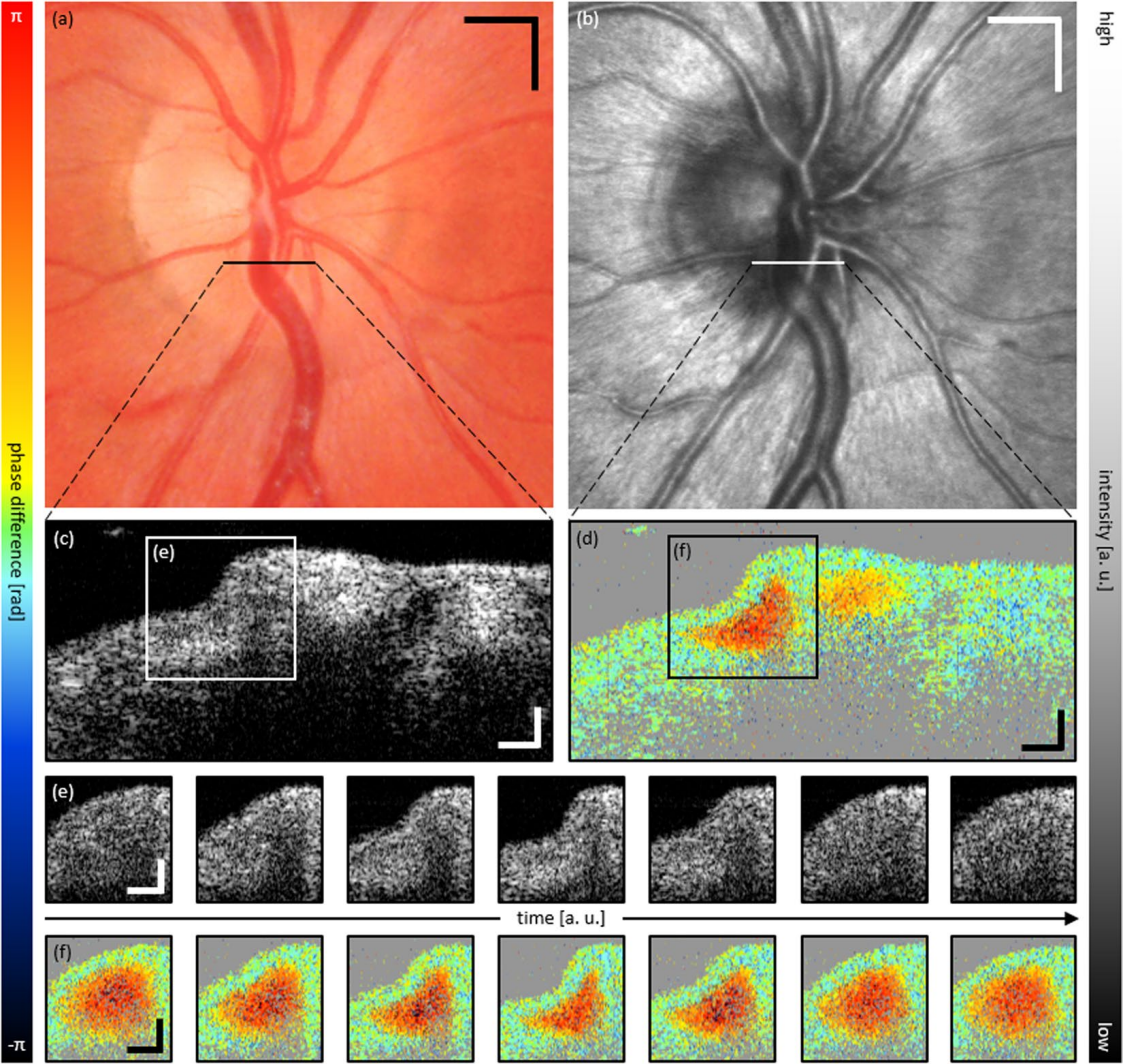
Representative multi-modal image data of SRVP of subject 1. (a) Fundus photography and (b) SLO image with indicated region of collapse. Cross-sectional OCT (c) intensity and (d) Doppler tomogram at indicated region of collapse. (e,f) respective time sequence over full cardiac cycle of ROI indicated in (c,d). Supplementary Videos S1 and S2 provide movie-files of the snapshot images of (b–d), respectively. Scale bars: (a,b): 0.5 mm; (c–f): 0.1 mm.
